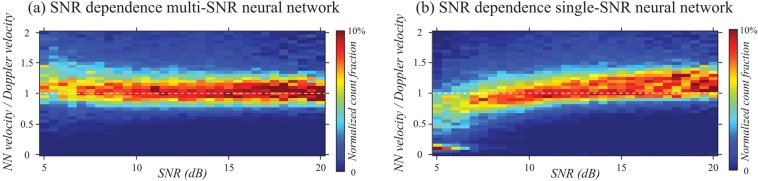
Using SNR-dependent NNs significantly reduces the SNR-bias in flow rate estimation. (a) Using SNR-dependent NNs, the distribution of the ratio of NN to Doppler flow velocity estimates are centered at unity for measurements SNRs from 5–20 dB. (b) Using a single NN trained at an SNR of 15 dB, the distribution shows a pronounced SNR bias.
